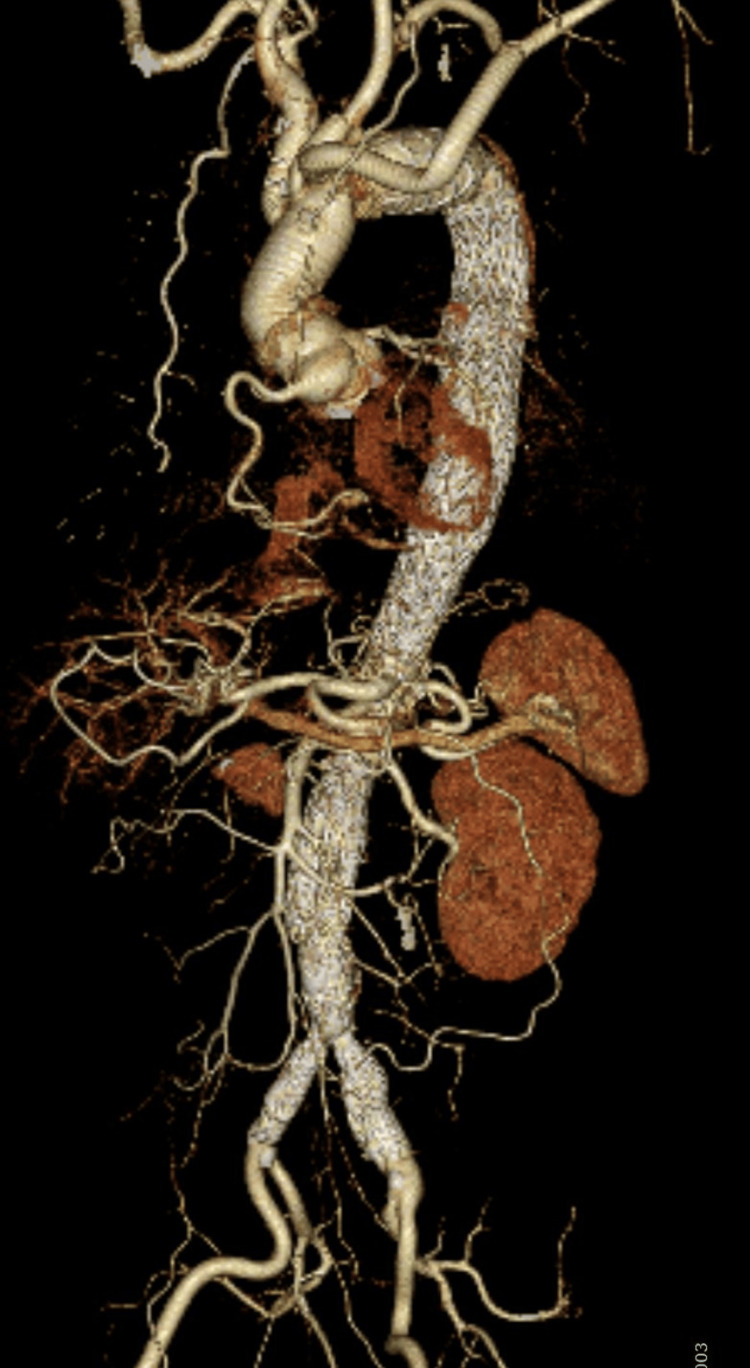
Postoperative whole-body contrast-enhanced CT No endoleak or residual aneurysm is observed. The thoracic and abdominal stent grafts are well-positioned, and the aortic morphology remains stable without any signs of migration, kinking, or sac enlargement.
